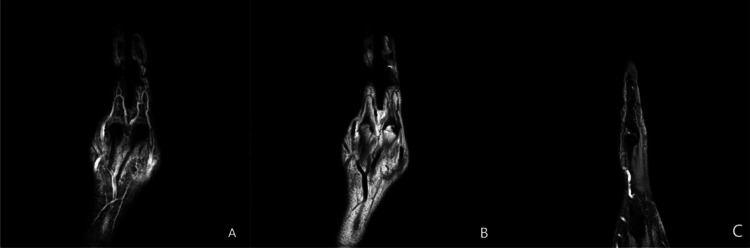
Follow-up magnetic resonance imaging (MRI) of the right hand on day 20 post-extravasation. (A) Coronal fluid-sensitive sequence (eg, T2-weighted/STIR) demonstrating resolving mild hyperintense signal (edema) primarily within the subcutaneous tissues of the dorsum of the hand. (B) Coronal T1-weighted sequence showing intact deep fascial planes and tendon structures without evidence of deep tissue necrosis or fluid collection. (C) Sagittal sequence confirming that inflammatory changes remain confined to the superficial soft tissues, with no signs of osteomyelitis or deep compartmental involvement.
